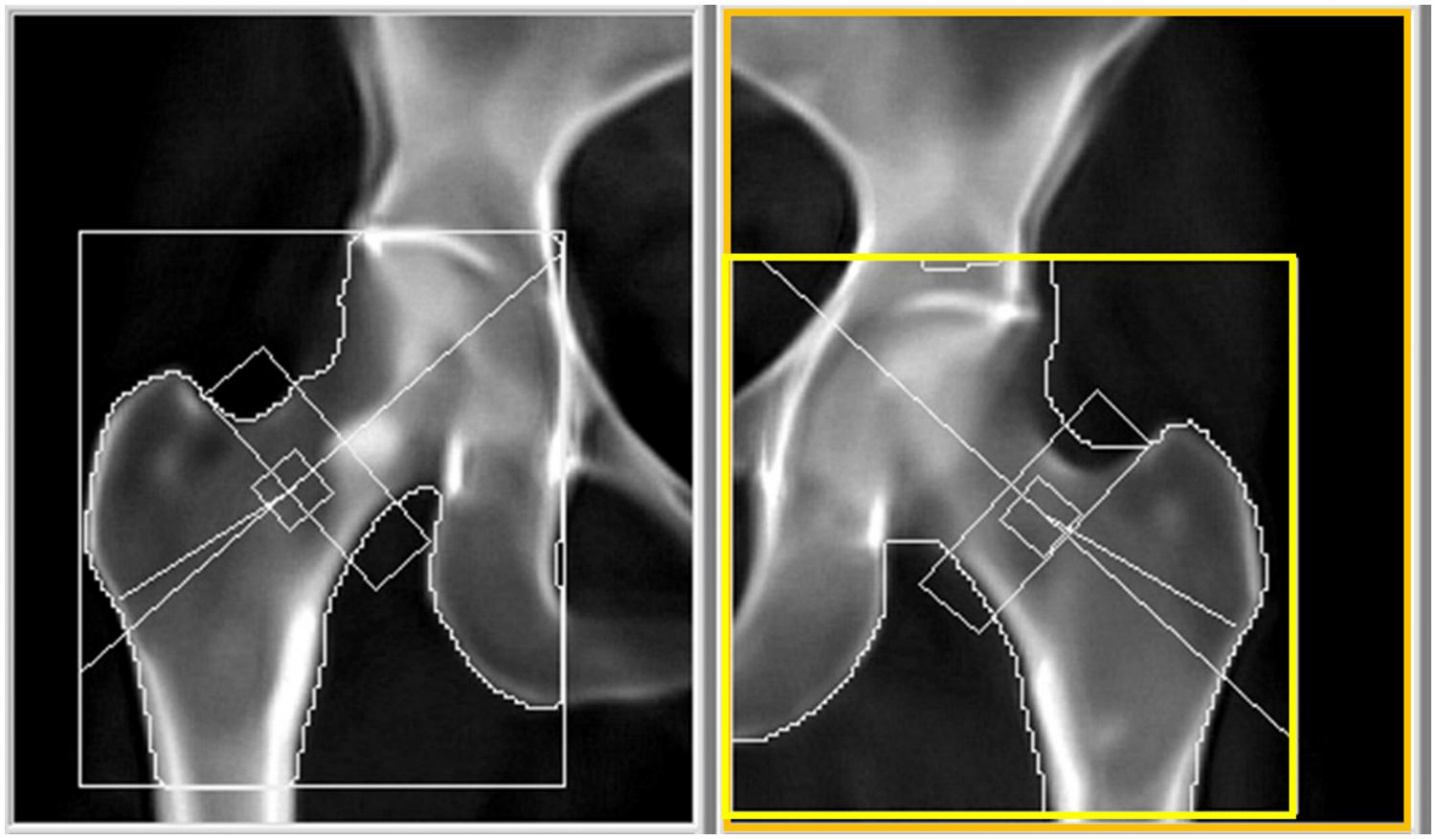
The initial DXA scan, the T-score of left femoral neck (right side) recorded as -6.8, while d_0_ was 270.3. DXA = dual-energy X-ray absorptiometry.
